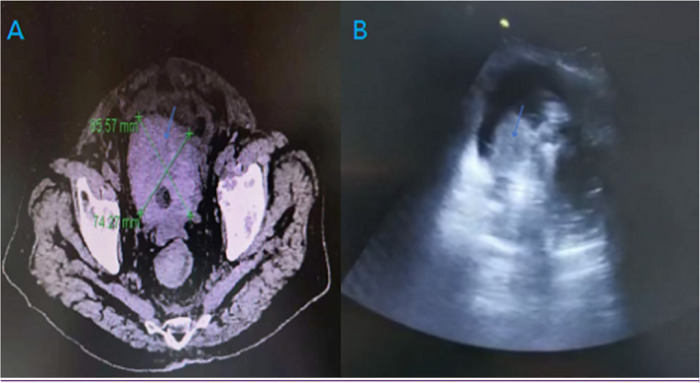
Imaging manifestations of large blood clot in bladder under CT scanning and ultrasound [(A): CT, (B) ultrasound; blue arrow: blood clot].
